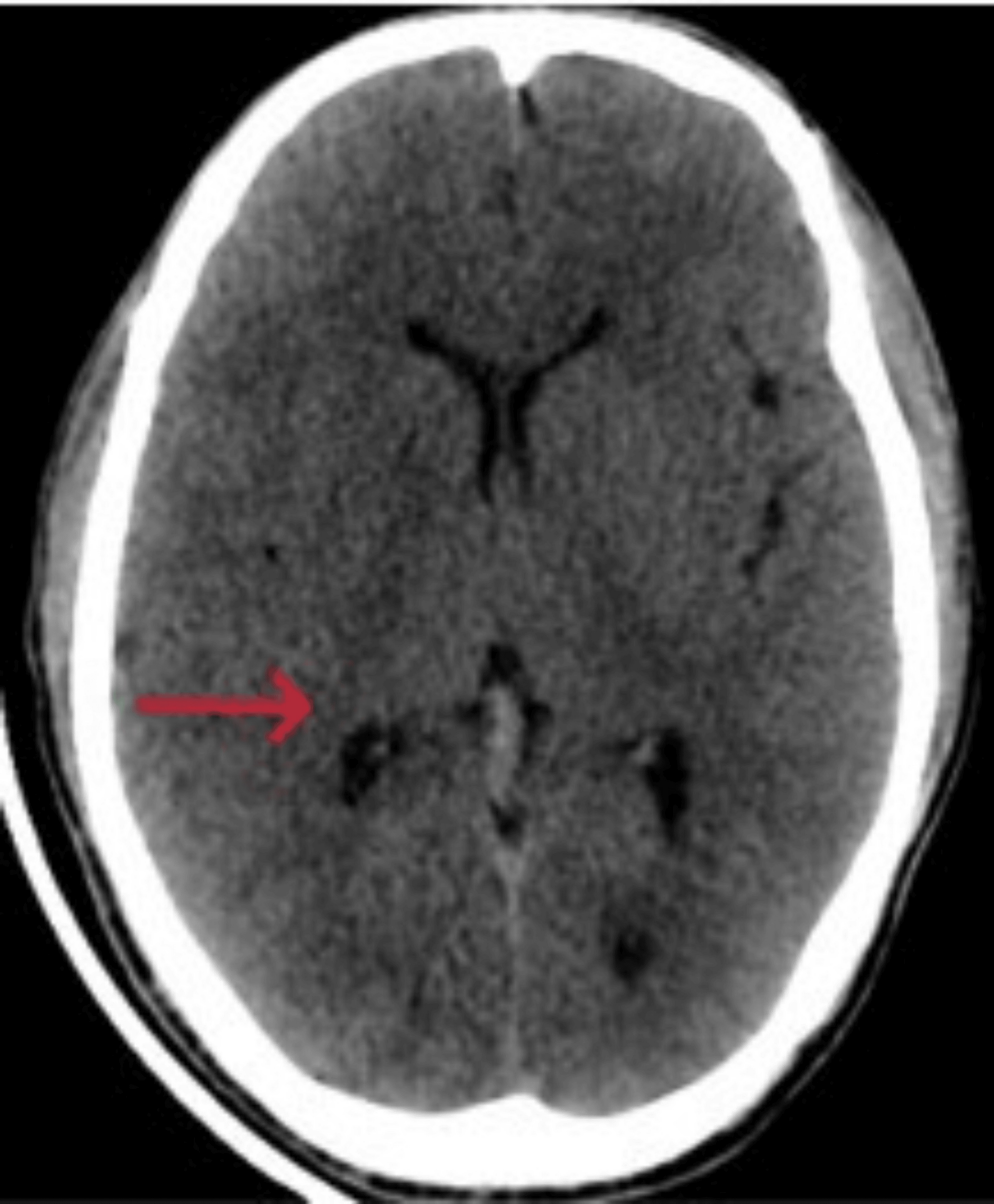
Trans axial CT scans passing through the third ventricle showing diffuse sulcal effacement indicating mild cerebral edema. CT: Computed tomography
